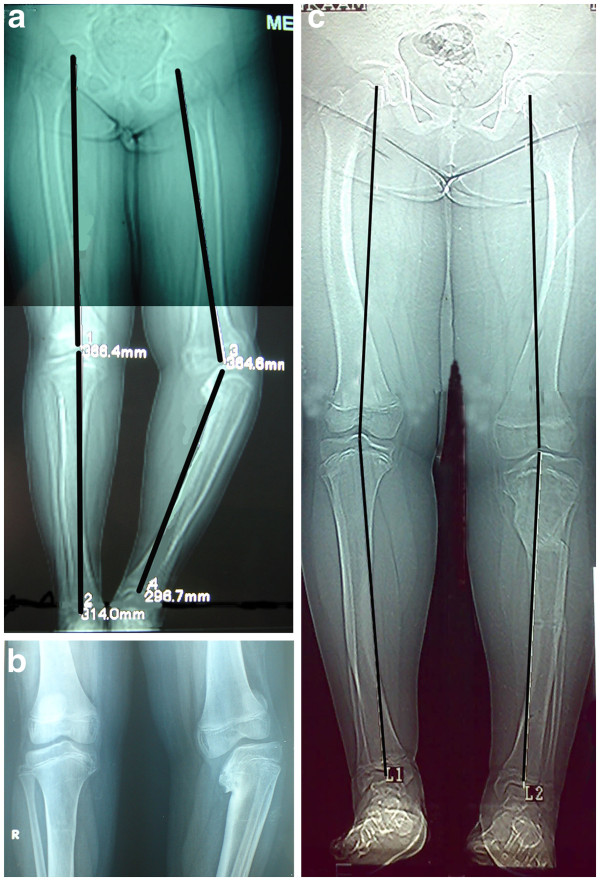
(a) Preoperative A-P radiogram of a 9 year old male with left recurrent late onset tibia vara with a preoperative DA angle of −45°. He had 25° of tibial internal tosion which was corrected acutely intraoperatively. (b) Roentgenogram of both knees shows left knee stage VI of the Langenskiold staging. (c) follow-up A-P radiogram after 4 years shows a recurrence of 15°.
